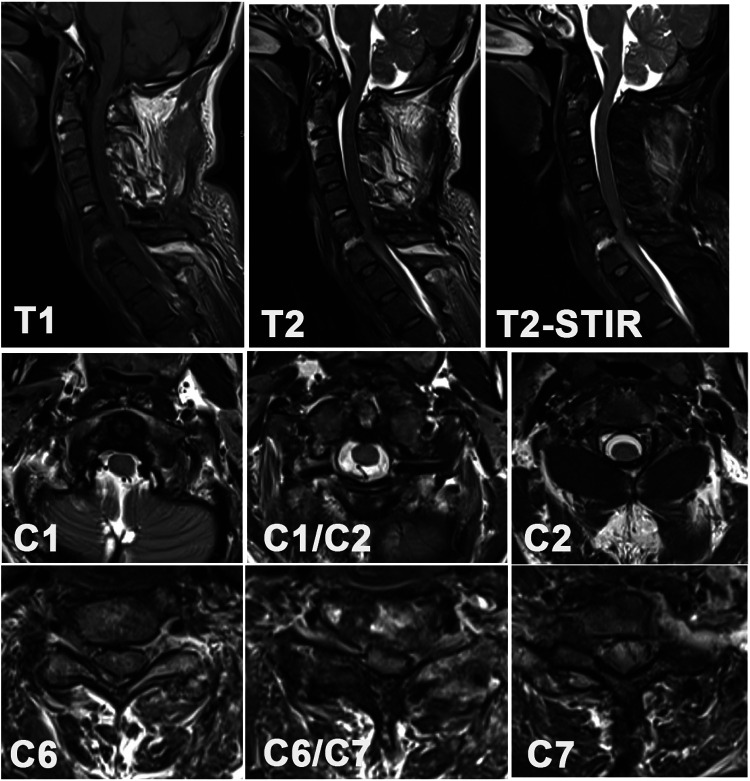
Preoperative cervical MRI.
